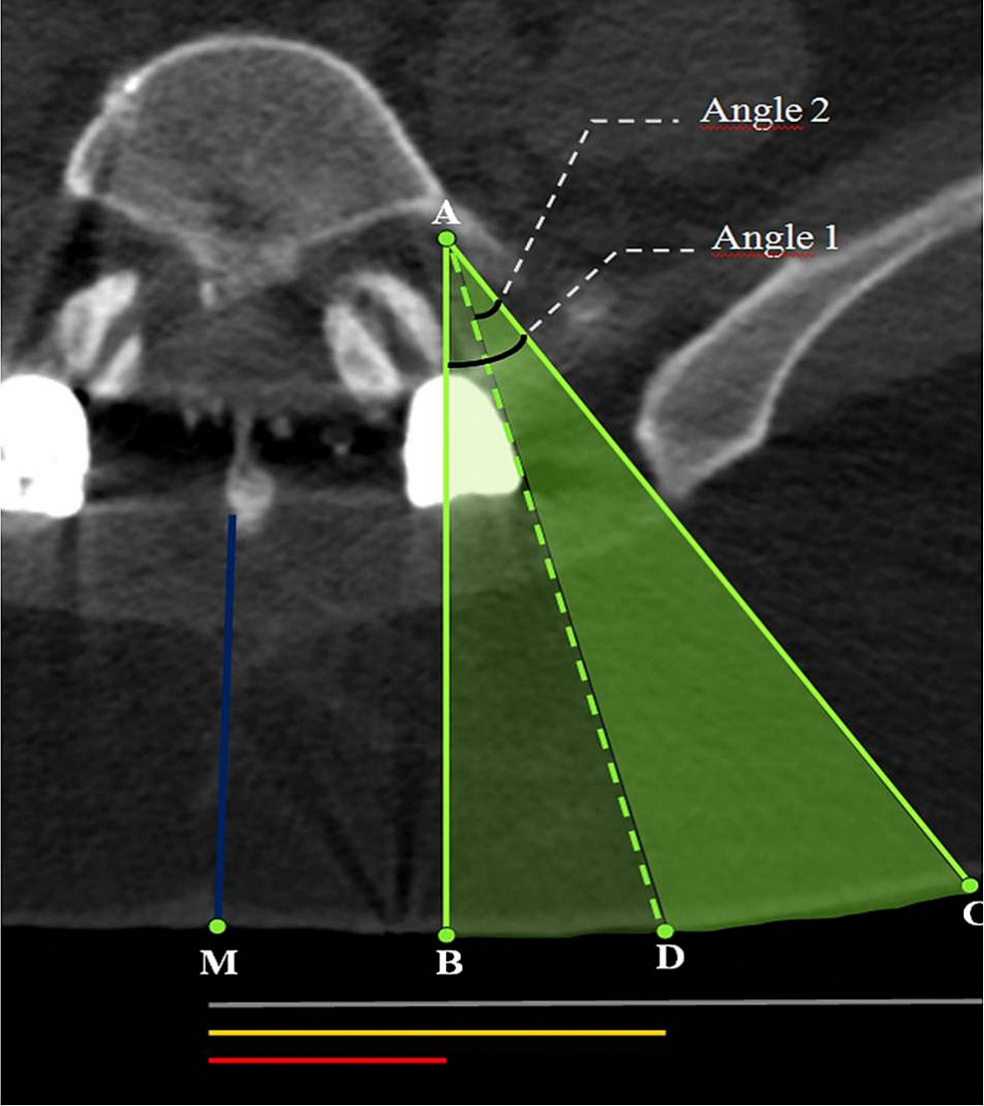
The measurements on axial lumbar computed tomography section. The lengths of the trajectories of A-B (shortest trajectory before transpedicular stabilization surgery), A-C, and A-D (shortest trajectory after transpedicular stabilization surgery) were measured. A, the target point for transforaminal nerve injection; B, The most medial entry point of the needle where transforaminal nerve injection can be performed before transpedicular stabilization surgery; C, The most lateral entry point of the needle where transforaminal nerve injection can be performed before transpedicular stabilization surgery; D, The most medial entry point of the needle where transforaminal nerve injection can be performed after transpedicular stabilization surgery; M: midline; Angle 1: the angle between the most medial and the most lateral trajectory where transforaminal nerve injection can be performed before transpedicular stabilization surgery; Angle 2:  the angle between the most medial and the most lateral trajectory where transforaminal nerve injection can be performed after transpedicular stabilization surgery. Gray, yellow, and red lines indicate the distance of the needle entry points from the midline.
